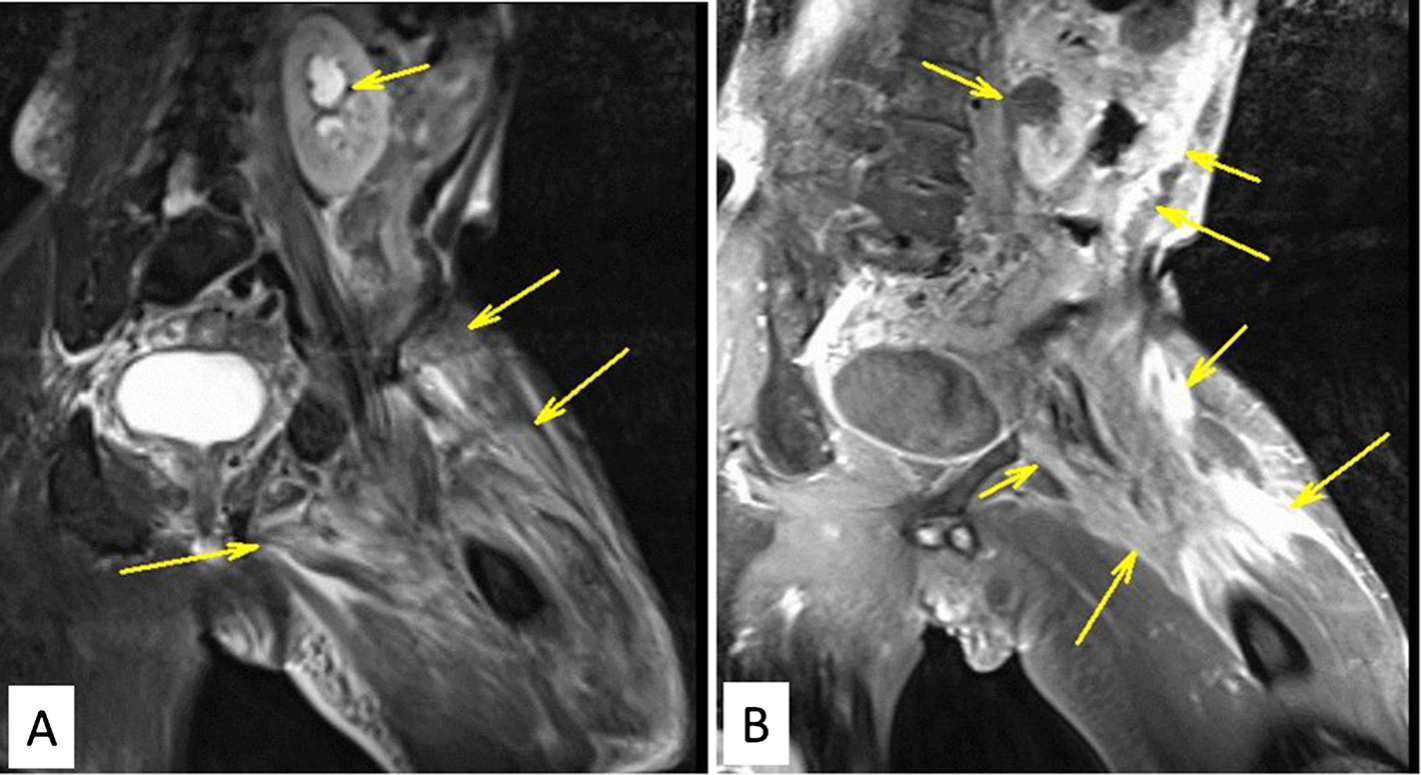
Magnetic resonance imaging (MRI) T1- and T2-weighted images. a MRI T2-weighted image showing the intramuscular mass has isosignal intensity. b MRI T1-weighted images showing heterogeneous intramuscular masses
